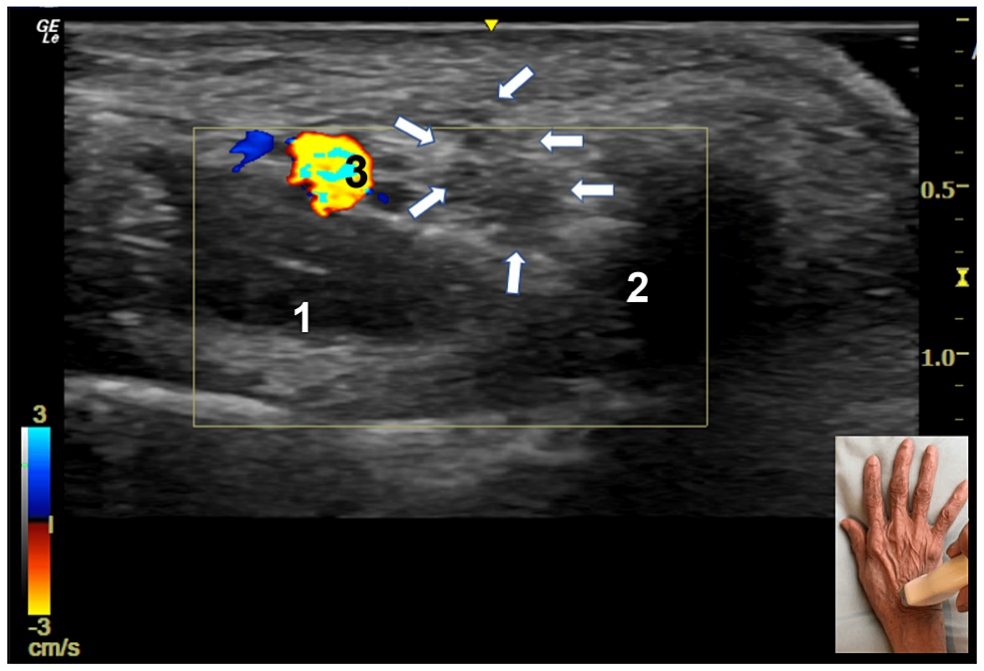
Ultrasound Study of the Distal Wrist Short axis view at the distal wrist showing the dorsal cutaneous branch of the ulnar nerve with prominent fascicles surrounded by hyperechoic tissue (arrows). The cross-sectional area of the DCBUN is 6 mm^2^ (normal ≤ 3 mm^2^). 1 and 2 are the 3rd and 4th dorsal interosseous muscles. A vein is depicted by 3. The scale for measuring the cross-sectional area is in mm^2^. DCBUN: dorsal cutaneous branch of the ulnar nerve
